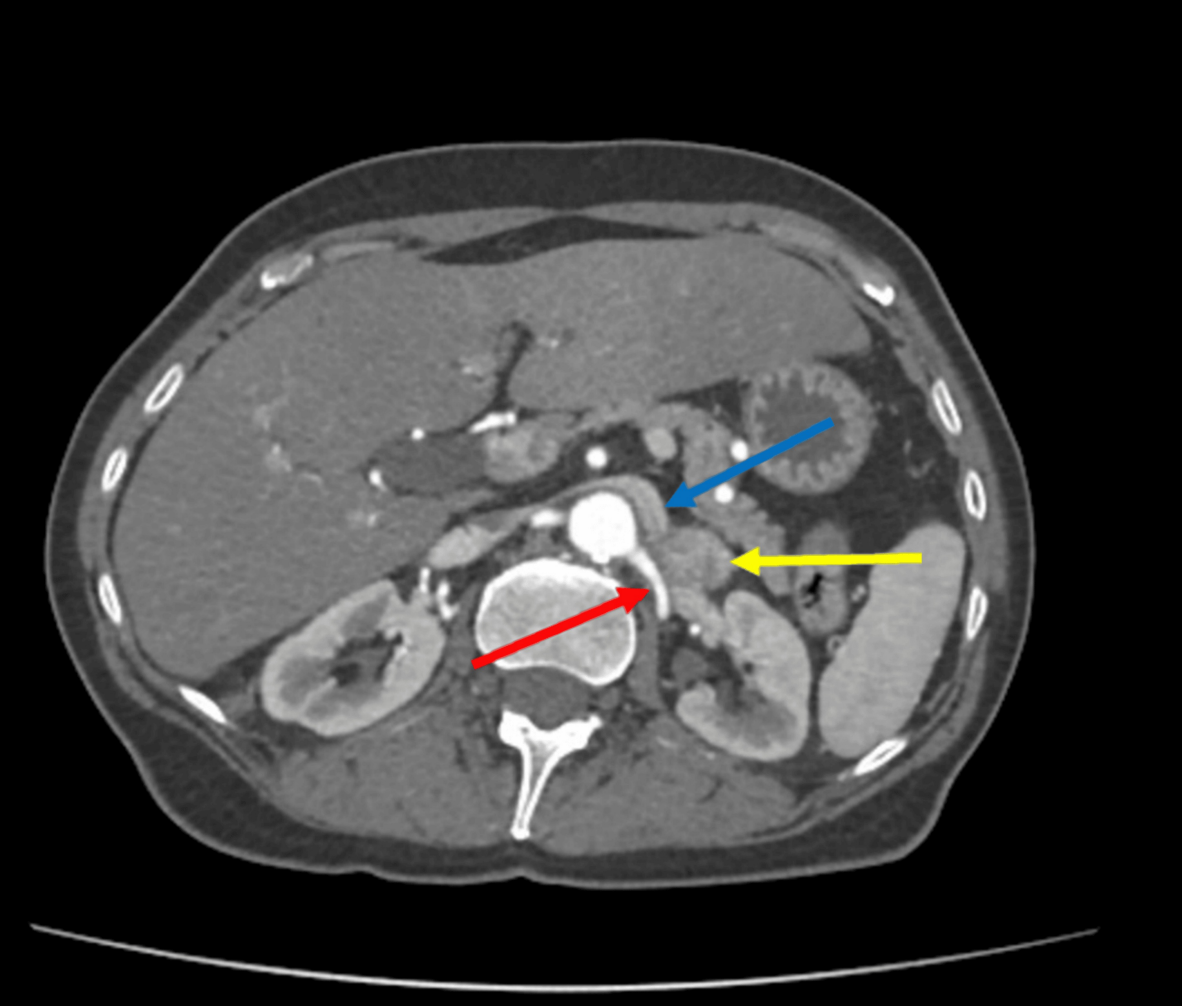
Cross-sectional CT imaging showing the retroperitoneal mass (yellow arrow) abutting the renal artery (red arrow) and displacing the renal vein (blue arrow) CT: computed tomography
